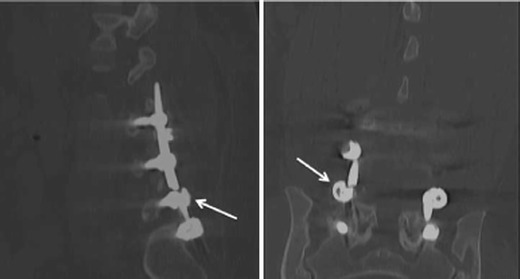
Sagittal and coronal CT reformats. Fracture of the vertical fusion rod with persistent anterolisthesis
